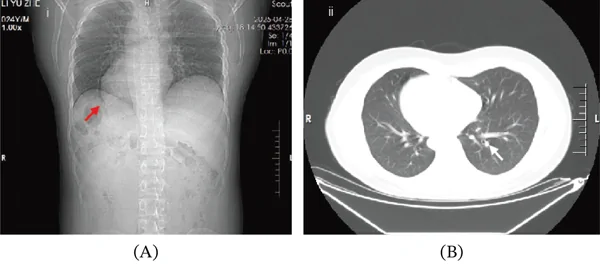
Radiological examination of the proband (IV:1). (A) The x‐ray (i) reveals typical dextrocardia (indicated by the red arrow). (B) The chest high‐resolution computed tomography (HRCT) (ii) demonstrates multiple cystic bronchiectasis, thickening of the bronchial wall, and signs of infection, manifested as multiple spot‐ and sheet‐like areas of increased density in both lung lobes (indicated by the white arrow).
